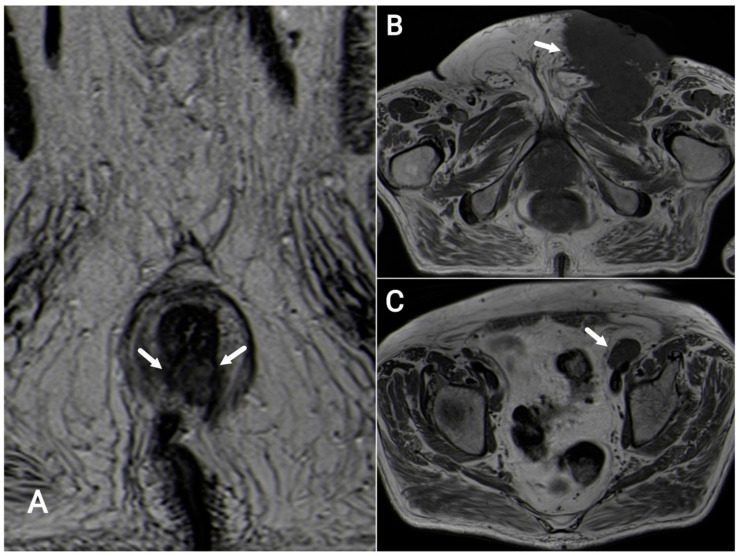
Squamous-cell carcinoma of the anal canal. Axial T2-weighted MR image (A, white arrows): 26 mm lesion from 5 to 7 o’clock invading the internal sphincter muscle bilaterally. Axial T1-weighted images: left inguinal adenopathy with cutaneous anterior extension (B); left external iliac adenopathy (C, white arrows).
